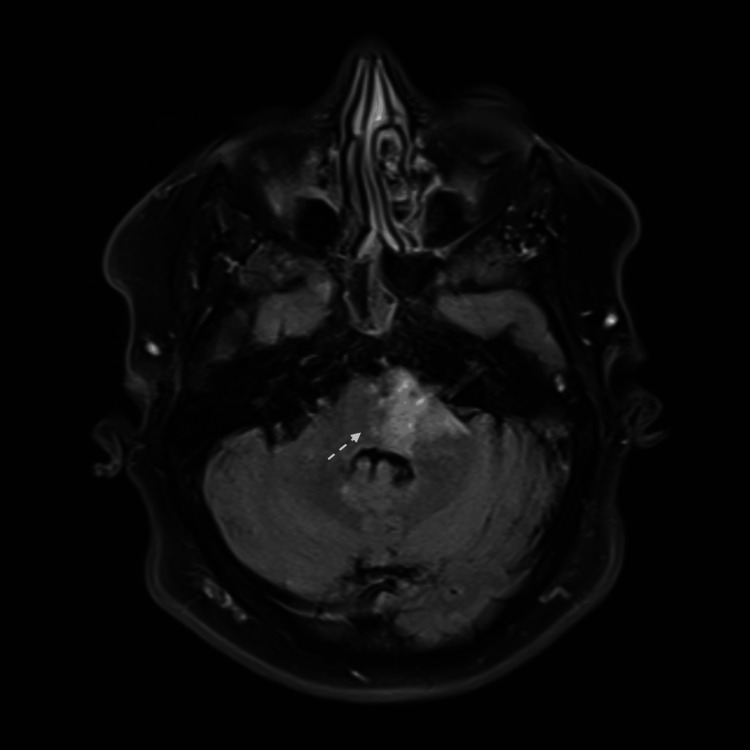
MRI brain FLAIR view showing left pontine hyperintense signal.
